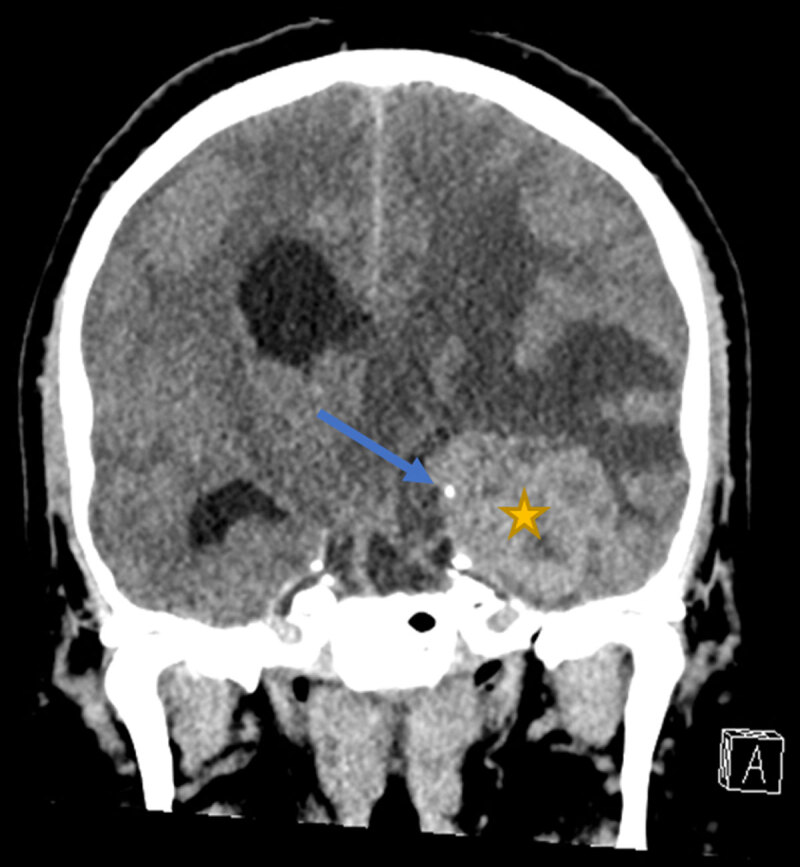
Subsequent magnetic resonance imaging (MRI) confirmed the presence of an extra-axial, dura-based, T2 hyperintense, T1 iso-intense, hypervascular mass (*Figure 2*, star) with large draining veins (*Figure 2*, blue arrow).
